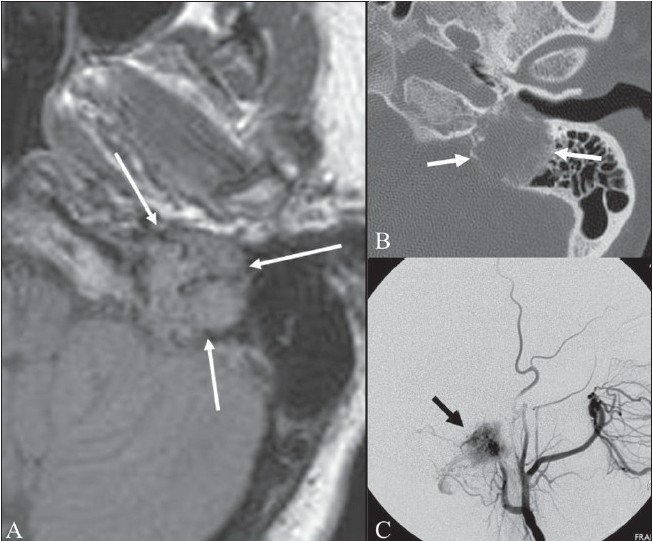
Salt and pepper sign. FLAIR axial MRI image of the left temporal bone shows an isointense mass (arrows) in the region of the jugular foramen. The mass is heterogeneous, with dark and slightly bright areas within it. Axial CT scan (B) in the same patient shows the mass causing destruction of the surrounding bone (arrows). An external carotid angiogram (C) shows tumor blush (black arrow) due to hypervascularity. These findings are suggestive of a glomus jugulare tumor of the jugular foramen
